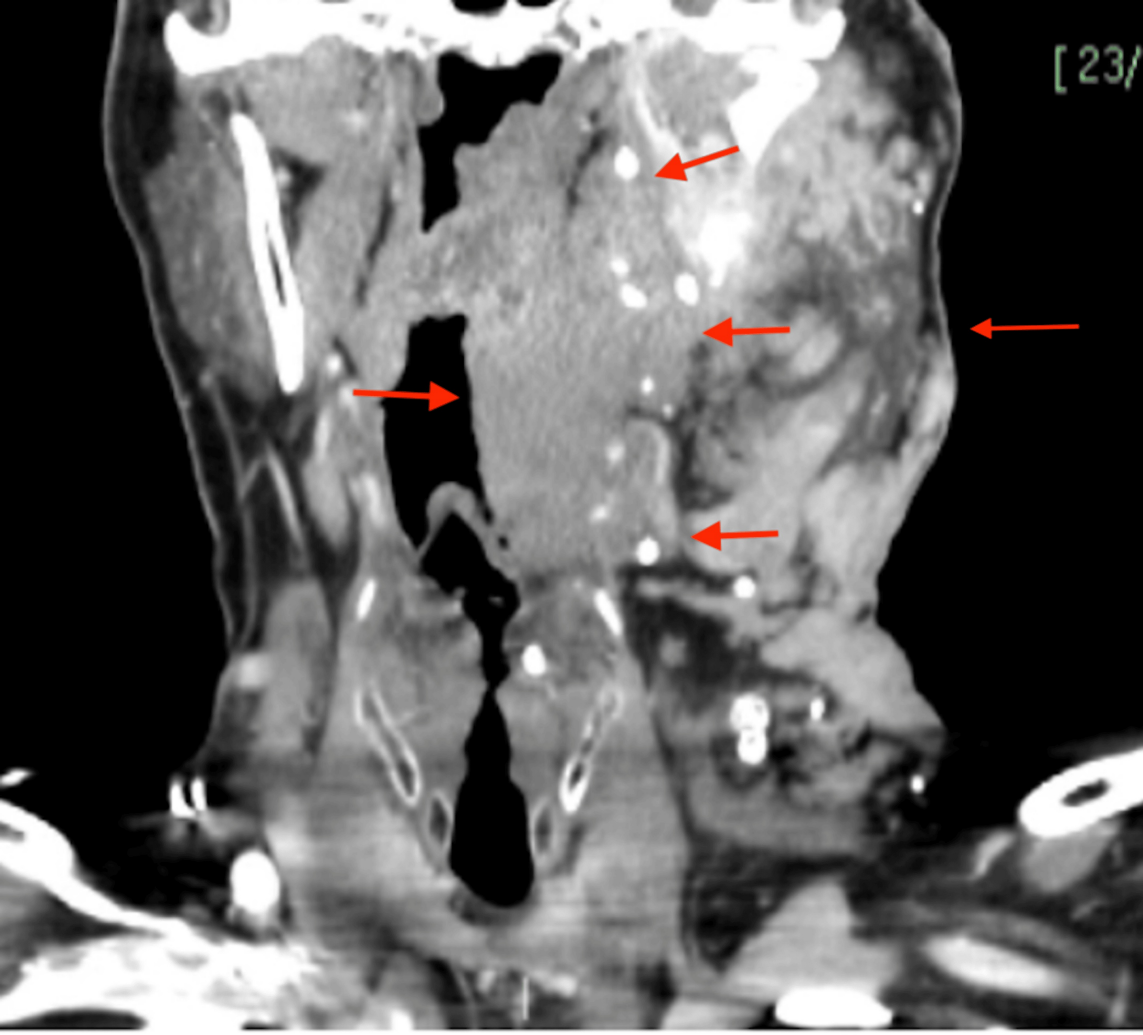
Coronal view of a CT scan of the neck at the level of the epiglottis demonstrating inferior and medial extension, as shown by the red arrows CT: Computed tomography
